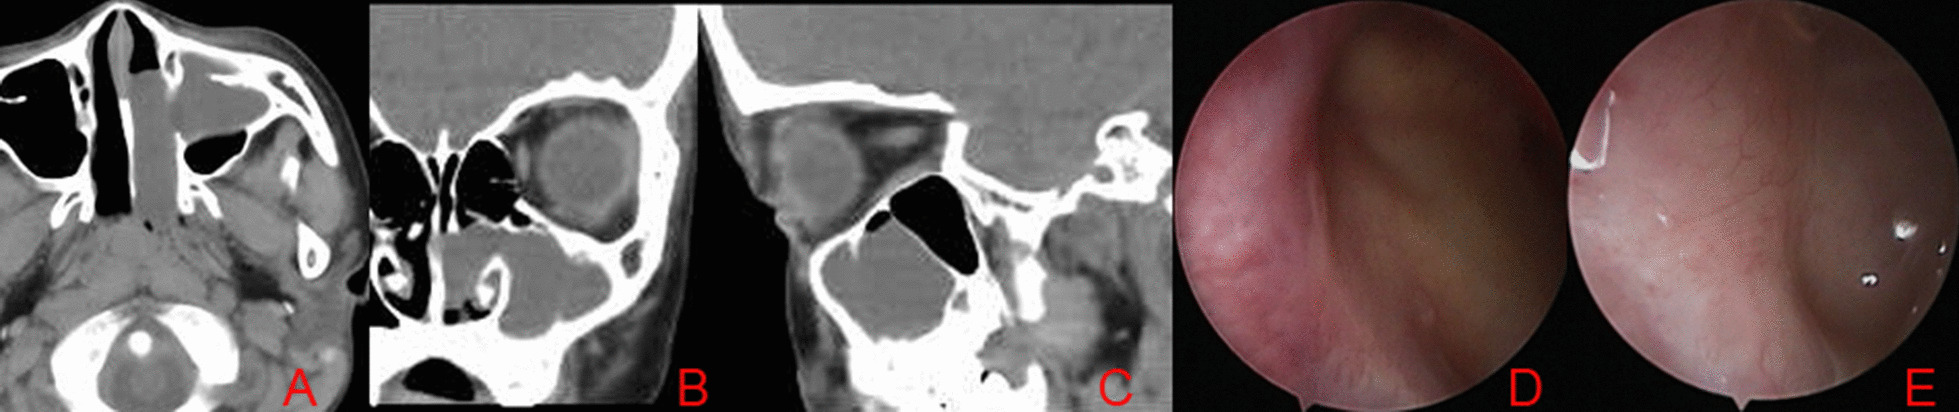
Preoperative CT revealed left ACP (A–C) in the patients with extended MMA, the maxillary sinus cavity via MMA at 6 months postoperatively (D) and well epithelization at 12 months postoperatively (E)
